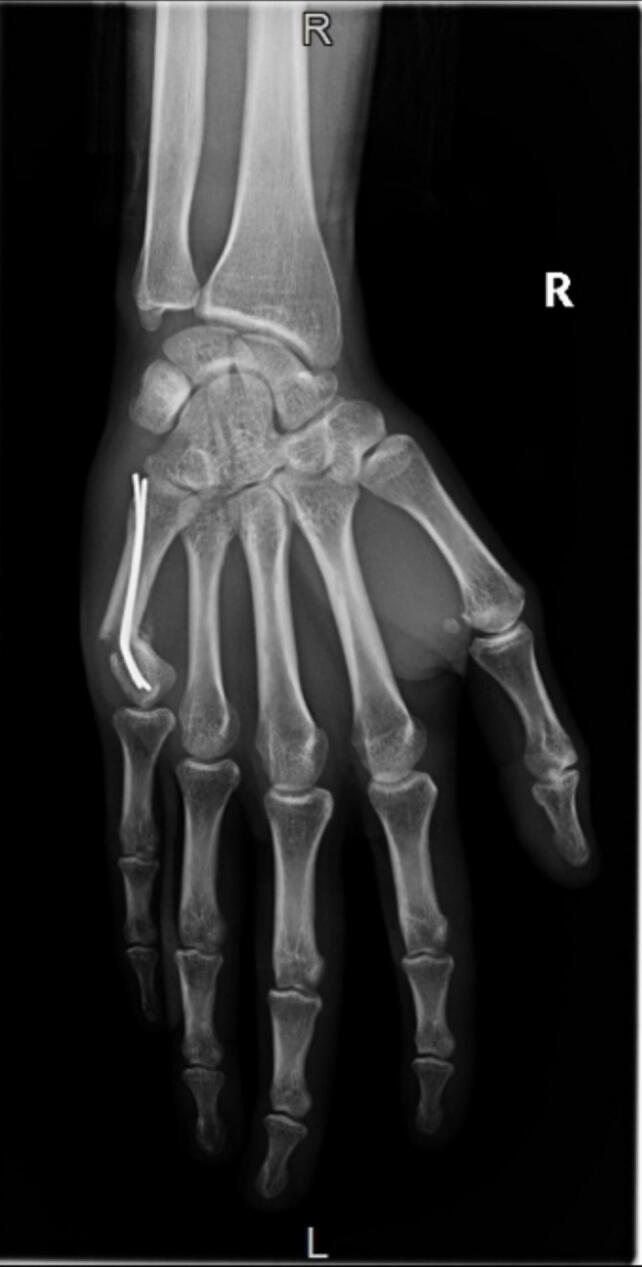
Preoperative X-ray showing bended pins in the fracture.
